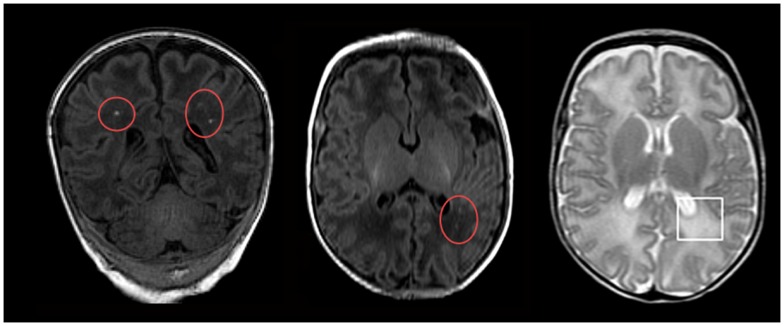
Magnetic resonance imaging (MRI) of neonatal hypoxic-ischemia. Neonatal hypoxic-ischemia is a common cause of white matter injury, the underlying factor in the development of neonatal seizures. These manifest on MRI as either punctate white matter lesions (red circles; seen on T1-weighted MRI imaging) or diffuse excessive high signal intensity (white square; seen on T2-weighted MRI imaging). Reprinted from Wisnowski et al. (2013) under the terms of the Creative Commons Attribution License.
